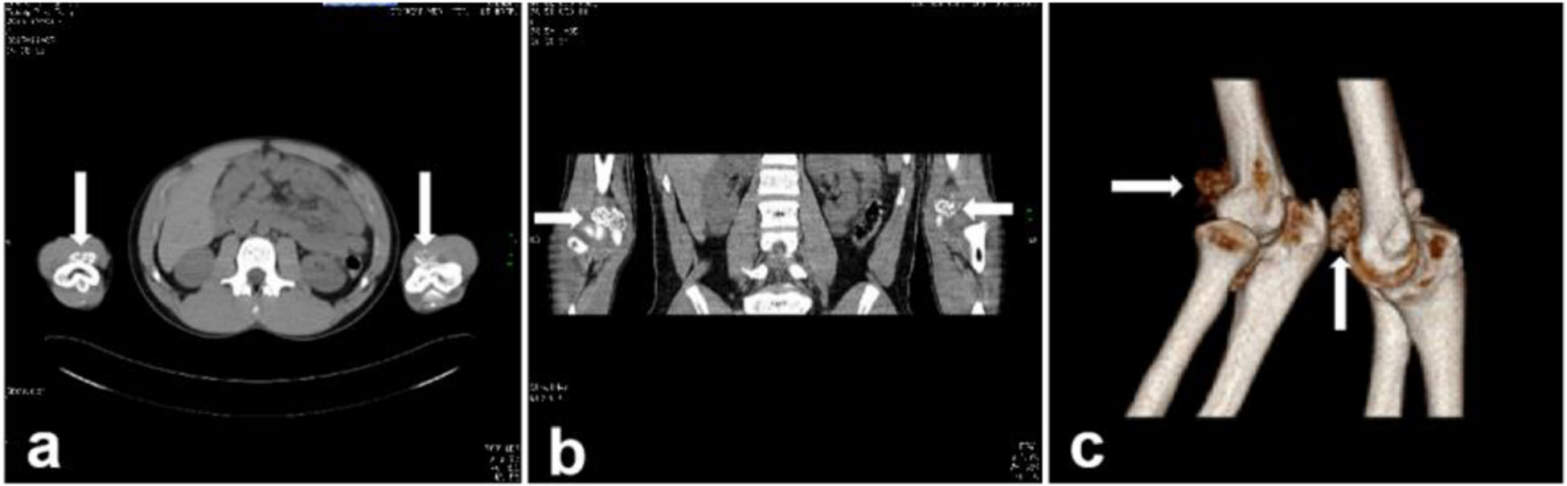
Computed tomography (CT) scan (a, transverse plane; b, coronal plane) and 3D-reconstruction (c) imaging of the bilateral elbows showed multiple calcified densities within the soft tissues surrounding anterior and posterior aspects of the elbow joints. Besides, there were sclerotic changes in the articular surface and the joints space were decreased. (arrow)
